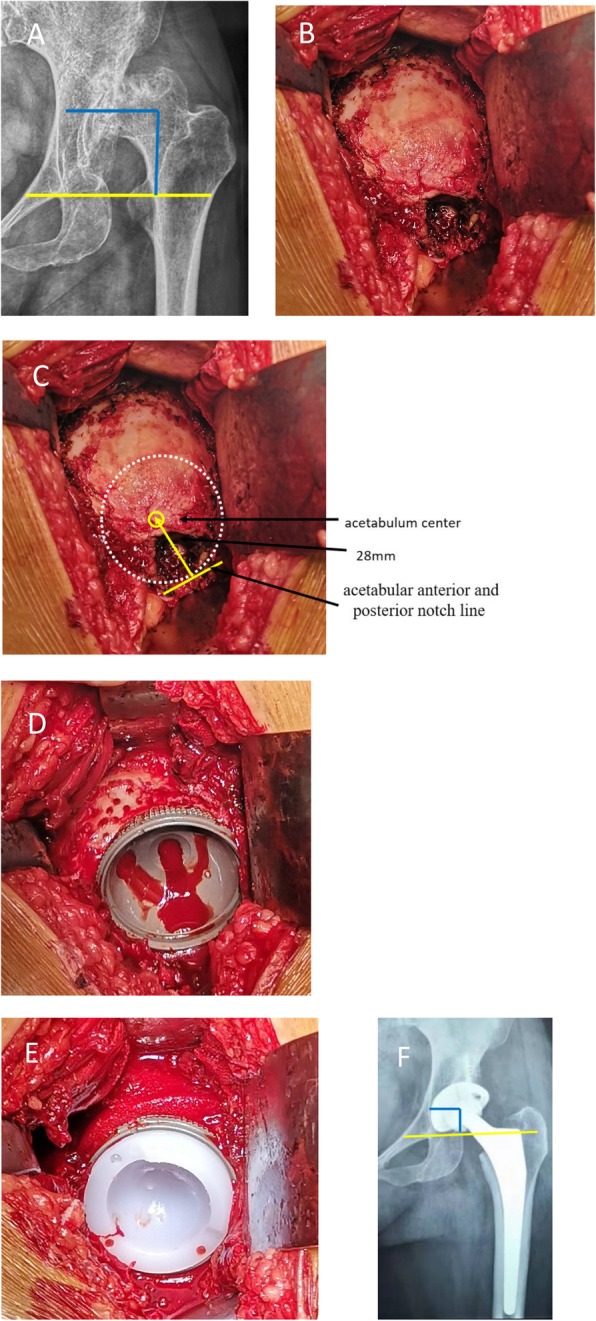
Radiographs of a 51-year-old female patient with end-stage osteoarthritis secondary to Crowe type III DDH. a Preoperative X-ray. b Intraoperative image showed the shell-shaped acetabulum and the triangle-shaped Harris fossa. c Locating the acetabulum center. d Reaming the acetabulum, installing the acetabular cup, and preparing the bone graft bed. e Installing the acetabular prosthesis and cancellous bone granule graft. f Postoperative X-ray and rotation center restoration
